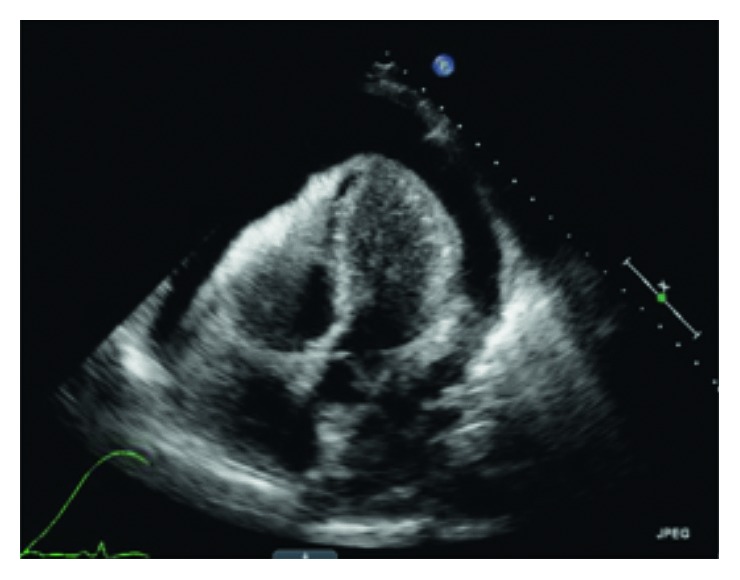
Echocardiogram showing large pericardial effusion with right atrial and ventricular collapse.
